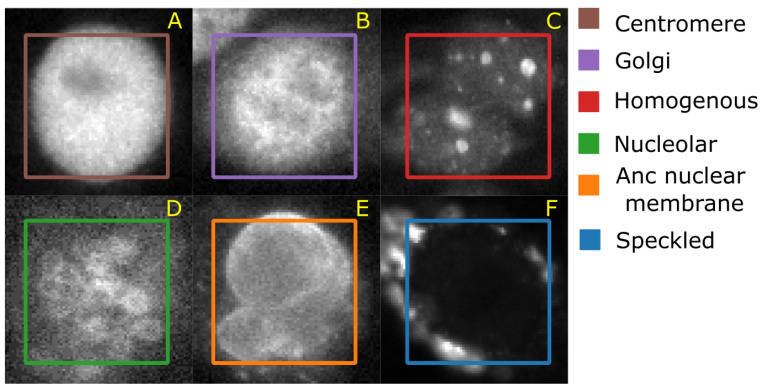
A representative image of each cell class and the average number of correctly classified instances in 10 cross validation. (A) Centromere (CT); (B) Golgi apparatus (GG); (C) Homogeneous (HM); (D) Nucleolar (NCL); (E) Anc nuclear membrane (ANM); (F) Speckled (SP).
